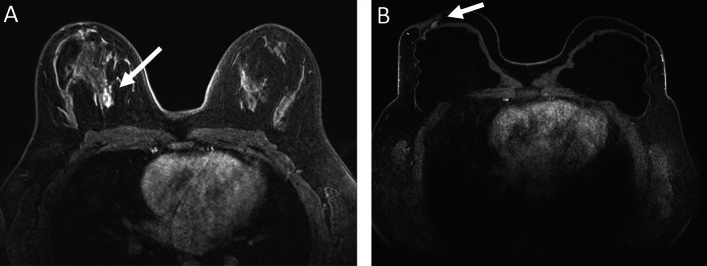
T1 gadolinium injected fat suppressed non-subtracted A MRI done at diagnosis, showing a tumour at upper central right breast, B MRI done at time of local recurrence, showing a tumour at the upper part of the right reconstructed breast, at the same axial level of the primary tumour
